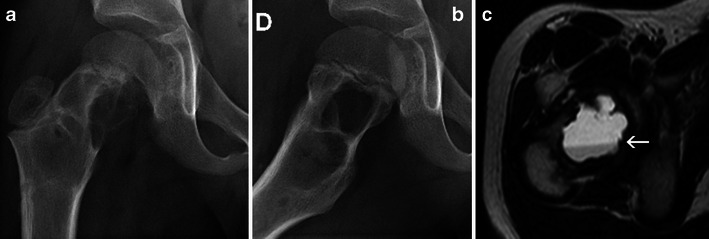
Simple bone cyst of the right femoral neck: Frontal (a) and lateral (b) X-rays of the right femur: well-defined central lytic lesion, with internal septa. Transverse T2-weighted MRI (c): a unique, large fluid-fluid level is identified (*arrow*)
